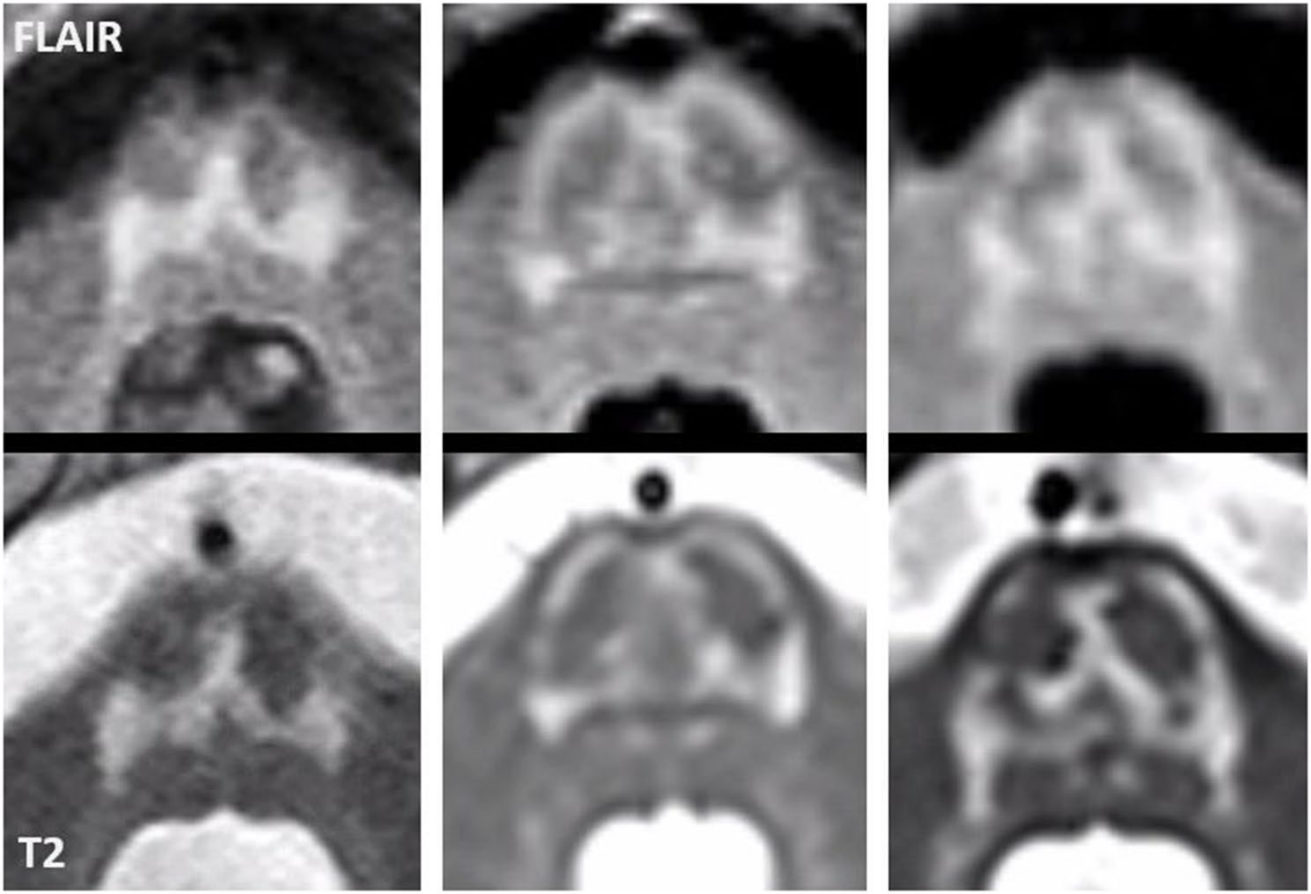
Cropped FLAIR and T2-weighted images of three different patients (from left to right: patient 3, 9 and 5) are shown, centered on the pons. Typical pontine gray matter involvement can be appreciated on both sequences, despite variation in quality of the images. Notice the sparing of the corticospinal tracts and the ascending sensory tracts, among which the medial lemniscus
